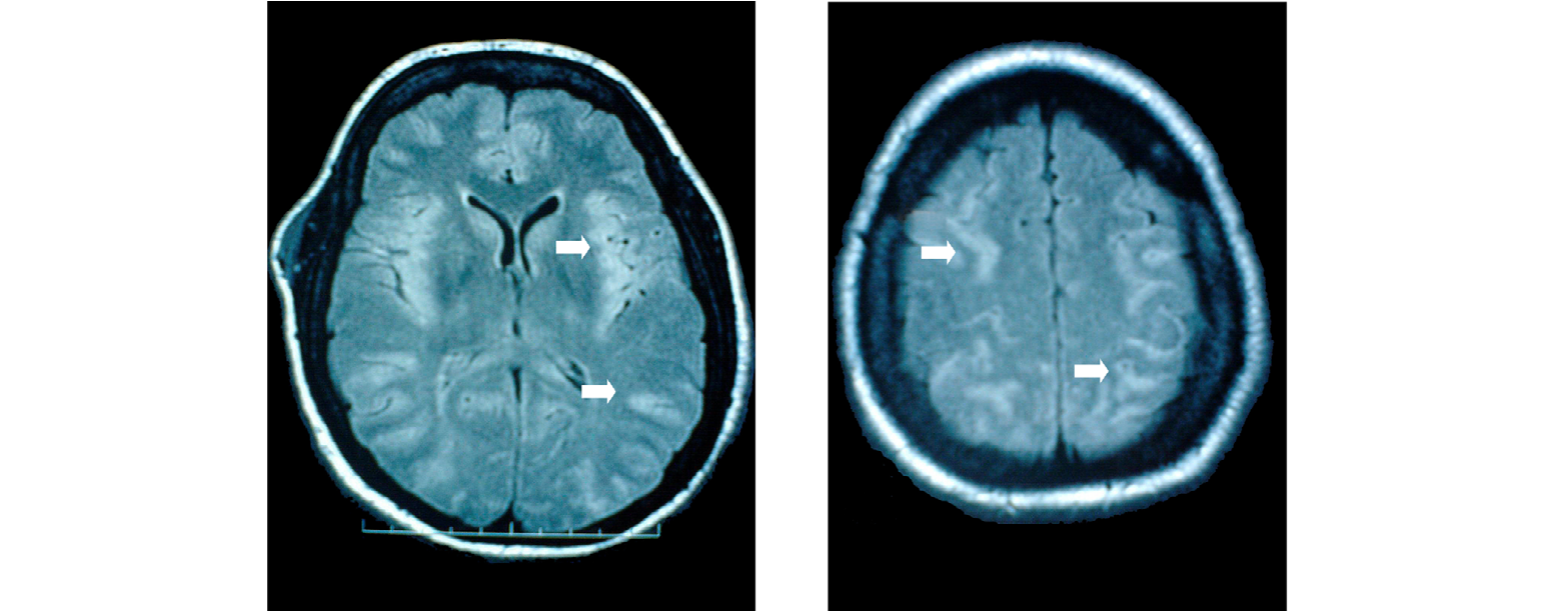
MRI findings in hypoglycemia-related encephalopathy. Cerebral fast fluid-attenuated inversion recovery magnetic resonance imaging (MRI) in a patient suffering from a severe hypoglycaemia-related encephalopathy on day 3 after deliberate insulin self-poisoning. The disseminated hypersignals of the cerebral gray matter (plain arrows) disappeared on day 30, whereas neurological impairments persisted.
